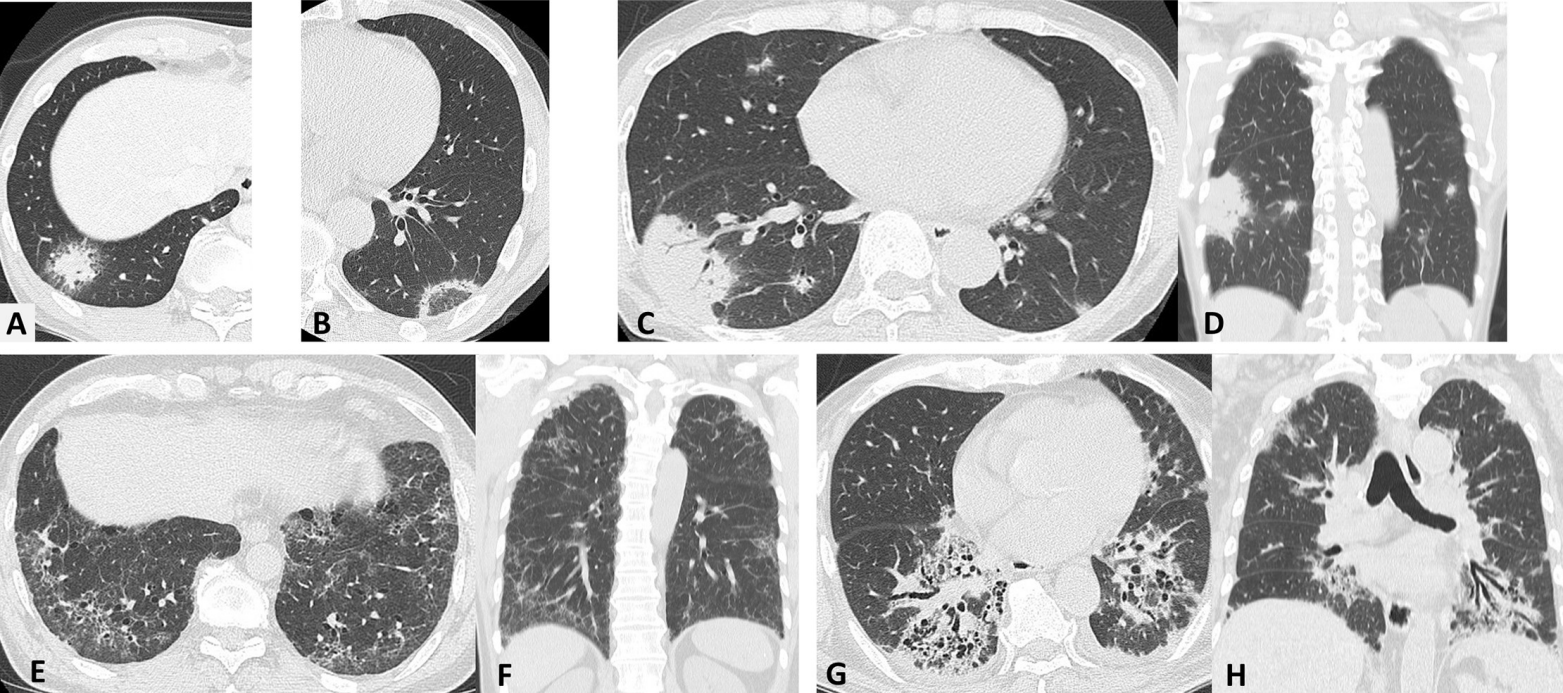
HRCT images of representative signs and CT patterns. (A) Halo sign: the nodular consolidation surrounded by GGO. (B) Reversed halo sign: rounded area of GGO surrounded by a ring of consolidation. (C, D) OP pattern: axial and coronal HRCT images showing patchy, peripheral predominant multiple consolidation with air bronchogram. (E, F) NSIP pattern: diffuse, subpleural predominant reticulation, and GGO with traction bronchiectasis. (G, H) NSIP and OP pattern: bilateral lower zone, peribronchovascular predominant consolidation, and GGO with traction bronchiectasis. HRCT, high-resolution computed tomography; CT, computed tomography; GGO, ground-glass opacity; OP, organizing pneumonia; NSIP, nonspecific interstitial pneumonia.
